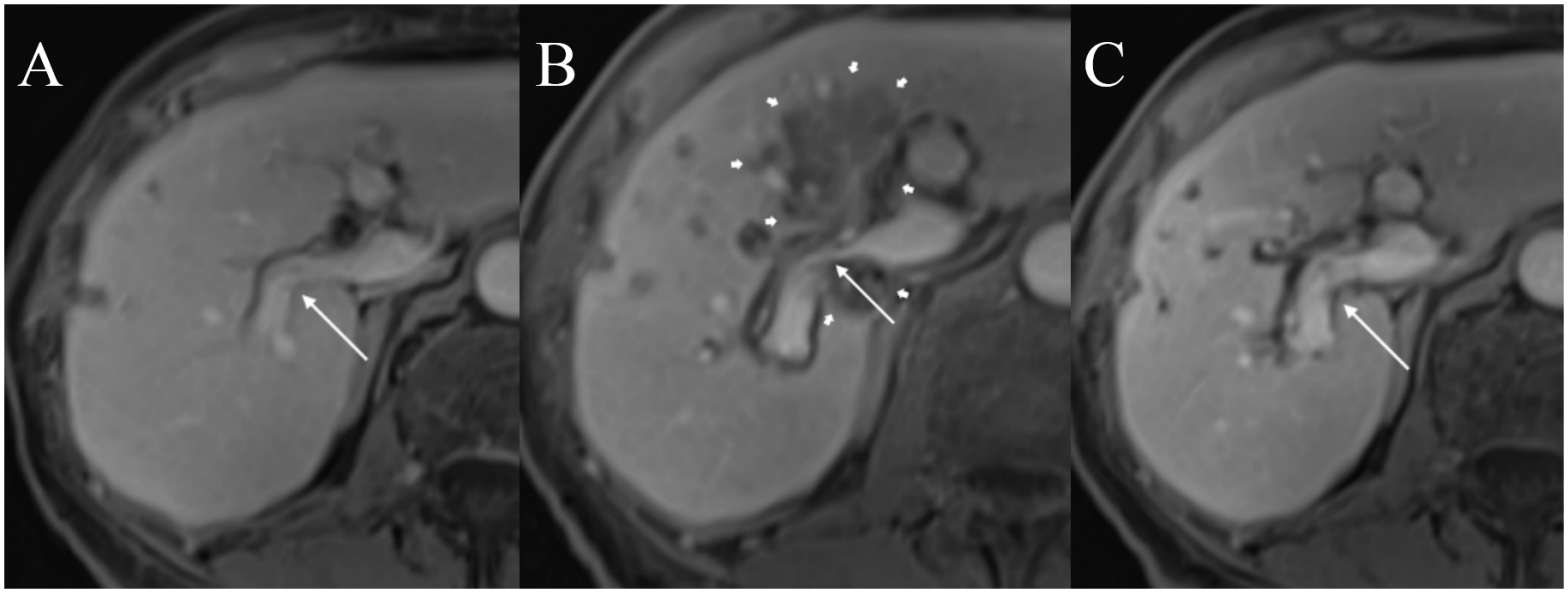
A 63-year old women (patient #4) with centrally located metastasis (not shown) of colorectal cancer. (A) Pre-interventional contrast-enhanced T1w magnetic resonance imaging (MRI) shows a freely perfused right branch of the portal vein (thin arrow). (B) A T1w MRI image obtained on the 3^rd^ post-interventional day shows a caliber reduction of the right portal vein (thin arrow) encased by the ablation zone (thick arrows). (C) 6 weeks after the intervention, the lumen reduction of the right portal vein has resolved (thin arrow).
